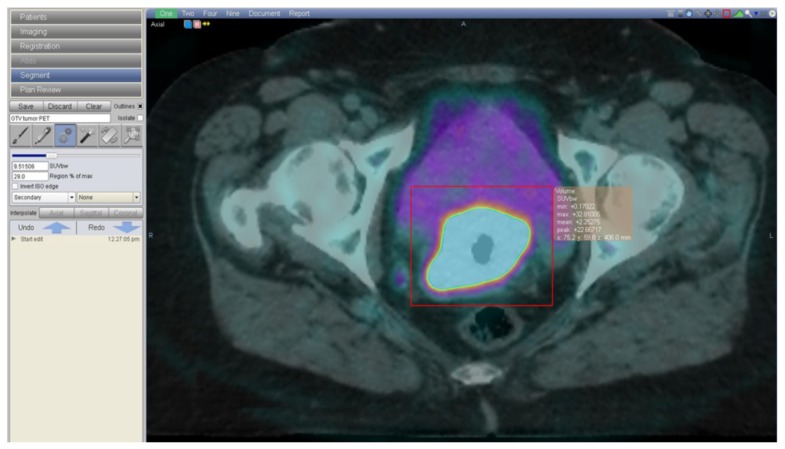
A 62-year-old woman with a FIGO IIB cervical cancer, treated with concomitant cisplatin based chemotherapy and radiotherapy. Image shows the radiotherapy contouring process on fused planning-CT and FDG-PET/CT images. Contouring is done on VelocityAI Software (Velocity, Atlanta, GA, USA), based on a method of automatic 3D volume segmentation of the functional image, that depends on the relationship between source to background ratio.
